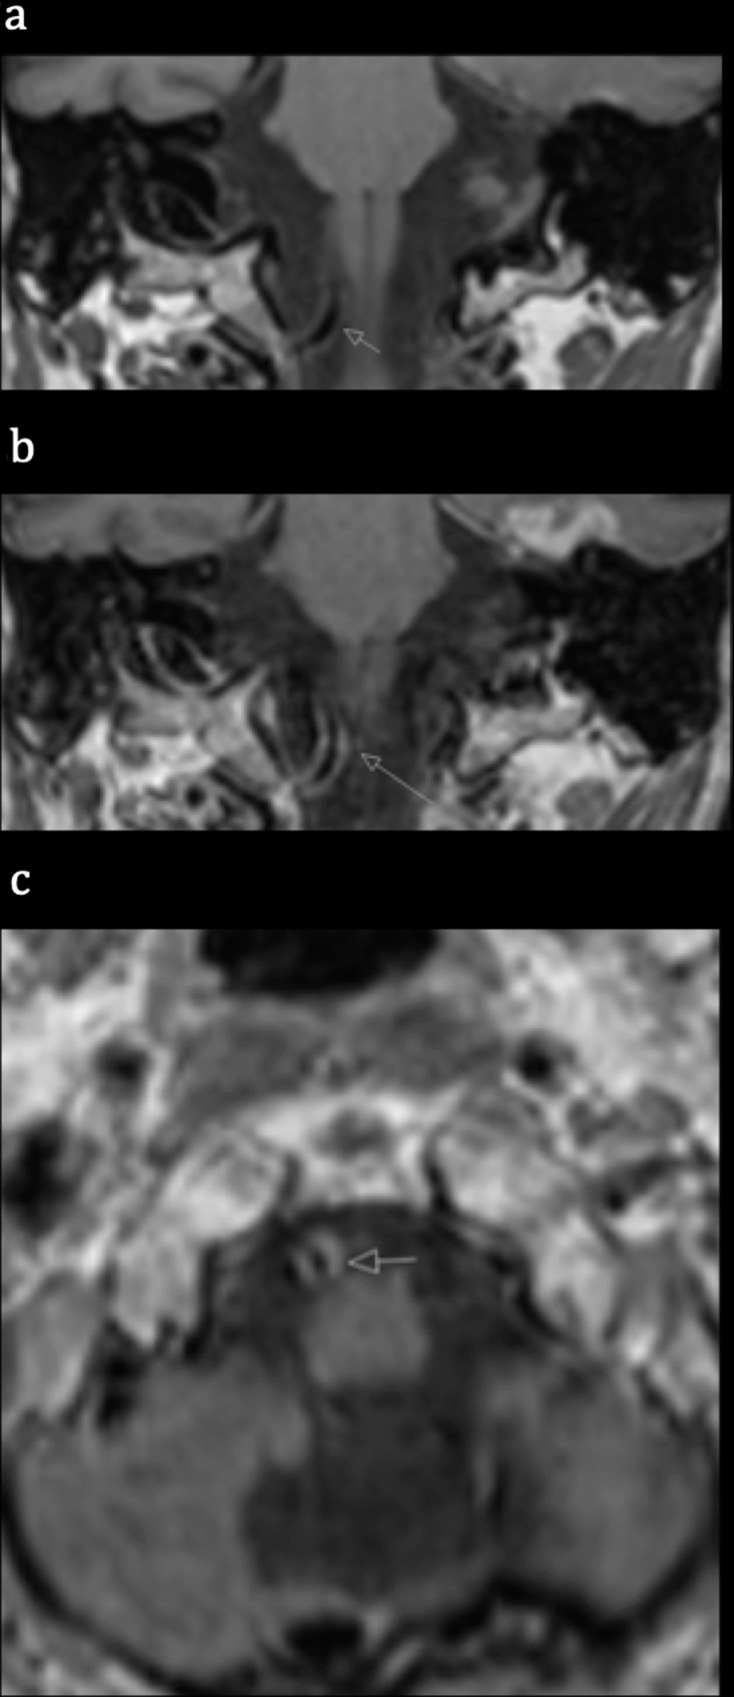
Blood suppressed vessel wall MRI coronal T1W pre-contrast (a), coronal T1W (b) and axial T1W (c) post-gadolinium demonstrating eccentric enhancement of the medial wall of the right V4 segment VA.
